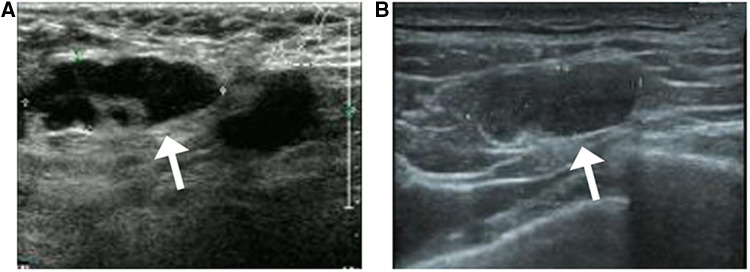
Sonographic findings from a 53-year-old patient with invasive ductal carcinoma with micropapillary features (A) axillary sonography showing multiple hypoechoic nodules in the affected axilla, the largest of which is approximately 2.9 cm × 1.1 cm in size, with poorly defined and irregular borders and poorly delineated corticomedullary structures (white arrow) (B) multiple hypoechoic nodules are visible in the left supraclavicular fossa, the largest of which measures approximately 1.8 cm × 0.6 cm in size, with poorly defined corticomedullary structures (white arrow).
